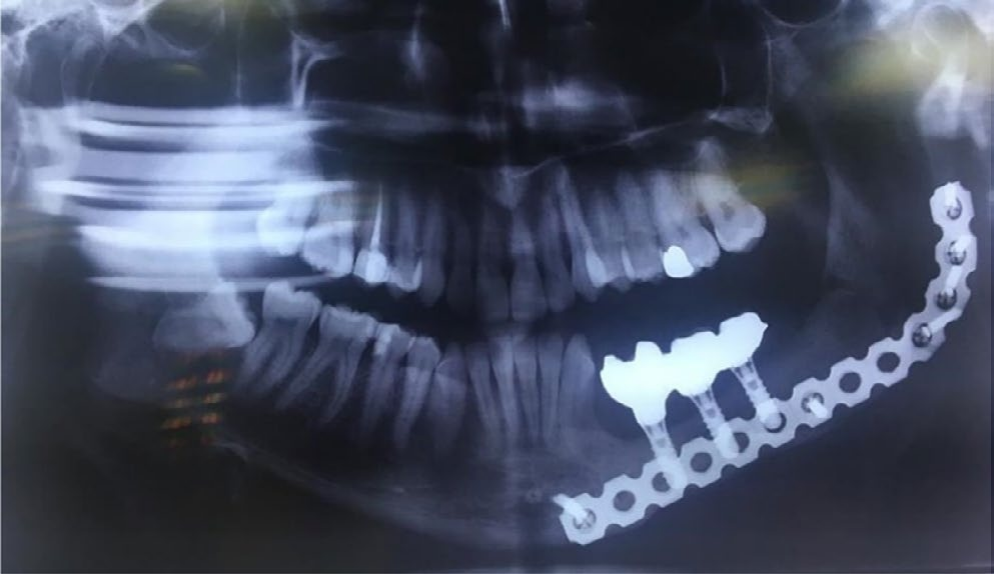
Delivery session radiograph
